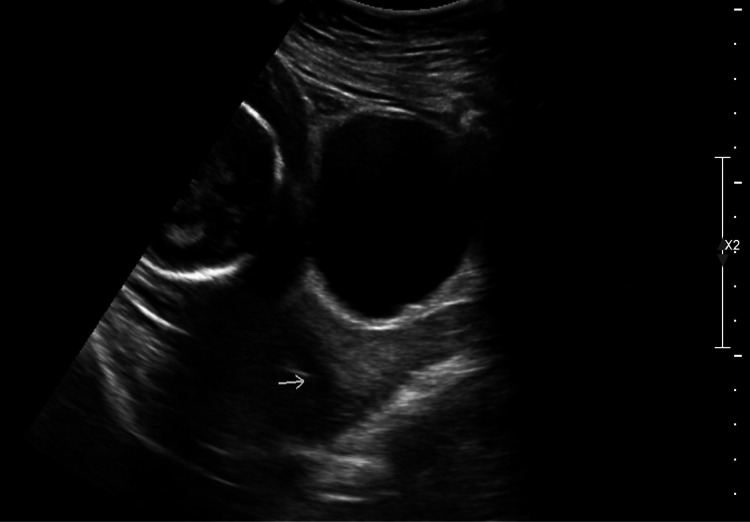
Transabdominal midline sagittal ultrasound image showing the presence of suture material in the endocervical canal after polypectomy.
